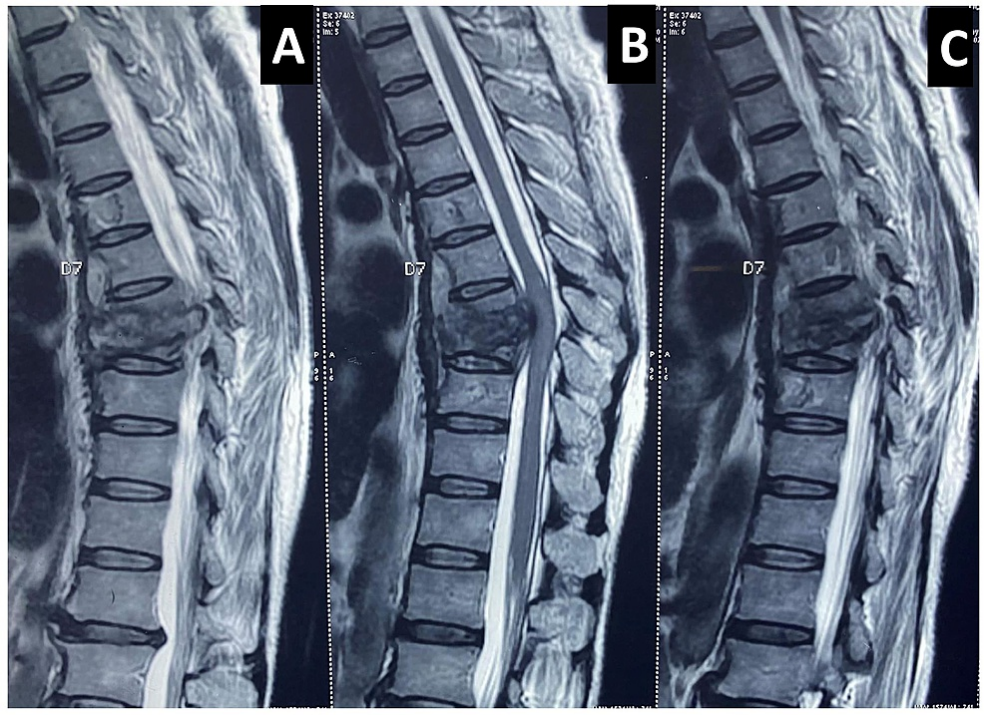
A, B, and C: Sagittal view T2-weighted MRI of the thoracic spine showing D8 vertebral body bony lesion, obliterated disc margins with anterior wedge necrosis. The vertebral body shows extensive tuberculous destruction bulging into the spinal cord with angulation of the bony column. D6, D7, D8, and D9 have central vertebral body lesions where the anterior longitudinal ligaments also look hypertrophied. The spinal cord at the D8 region looks compressed, but no edema or signal intensity changes were noted. There is no pre- or paravertebral cold abscess or gibbus formation. Discs, pedicles, and lamina are well preserved.
